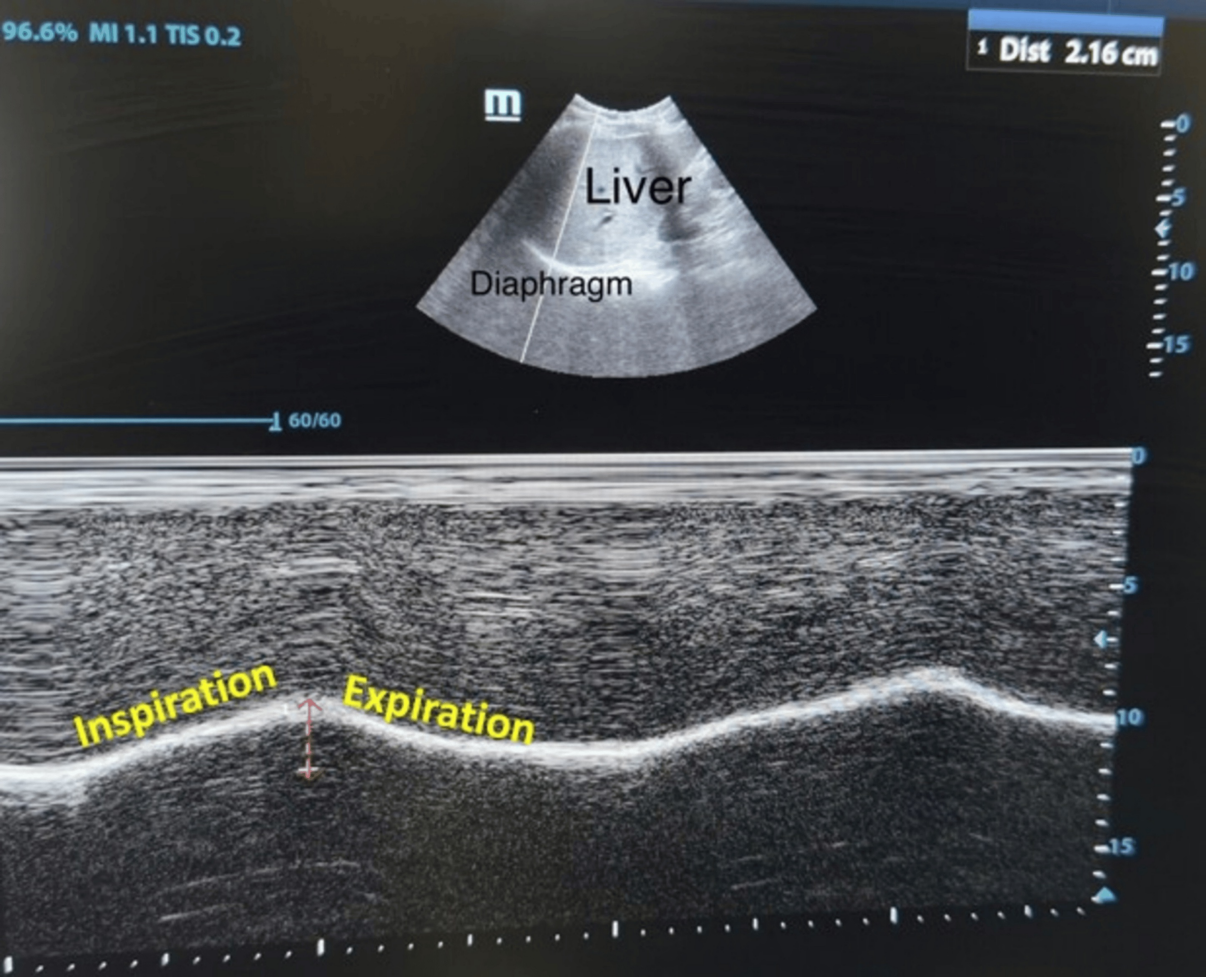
Right diaphragmatic excursion measured using M-mode sonography with the curvilinear probe.
